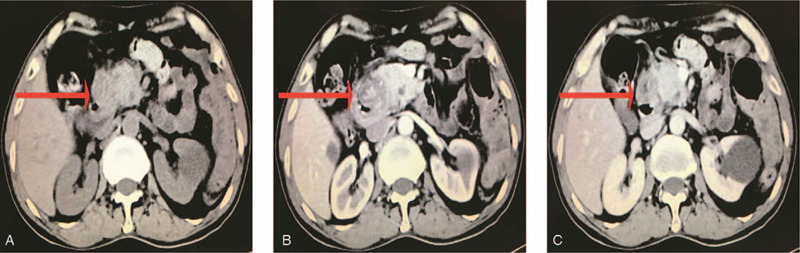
Conventional and enhanced CT images. Large (4.5 × 5.5 cm diameter) hypervascular exophytic mass in the Duodenum in CT (red arrow head).
